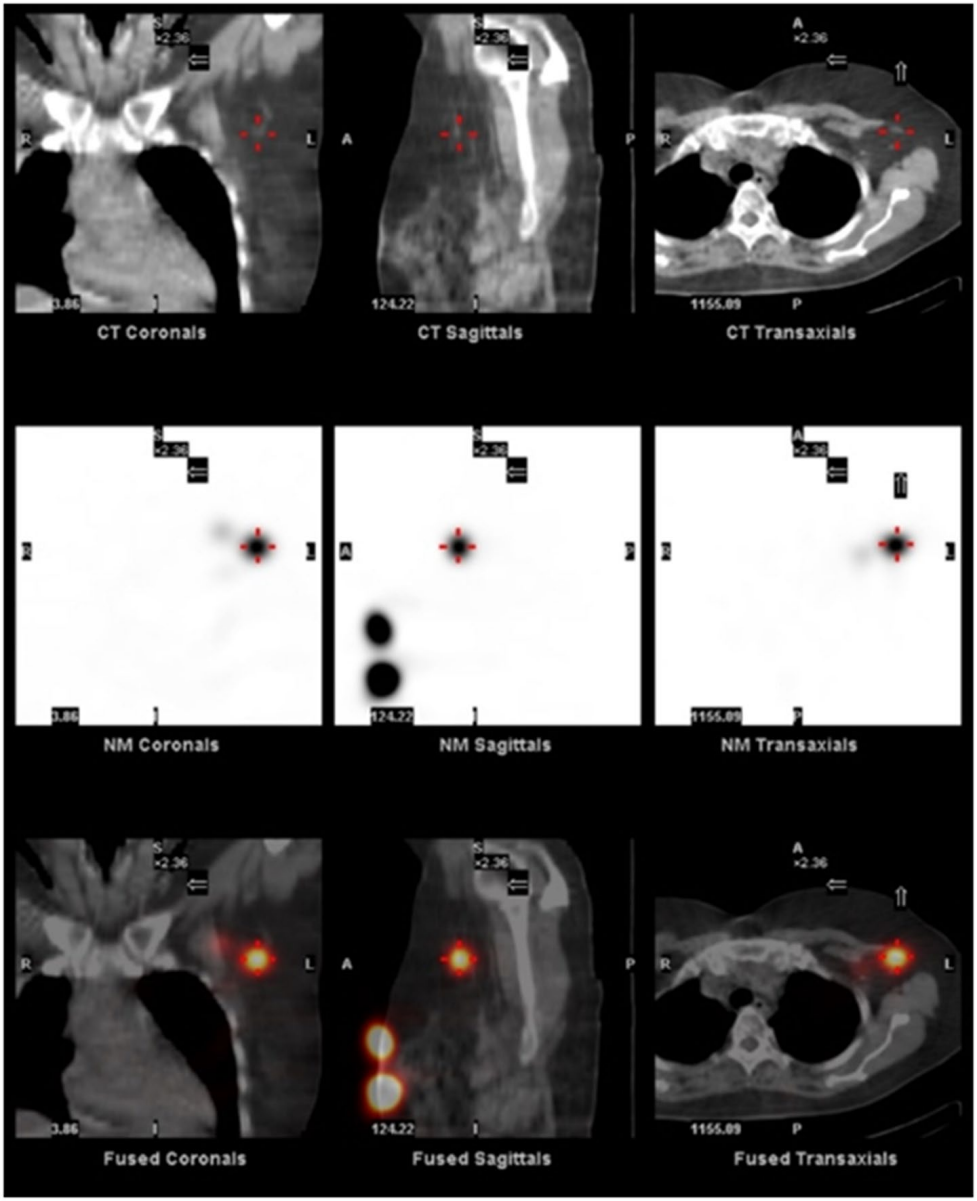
Example SPECT/CT images from a breast cancer patient showing a SLN annotated with a red cross in the axilla level I (anterior) node field
